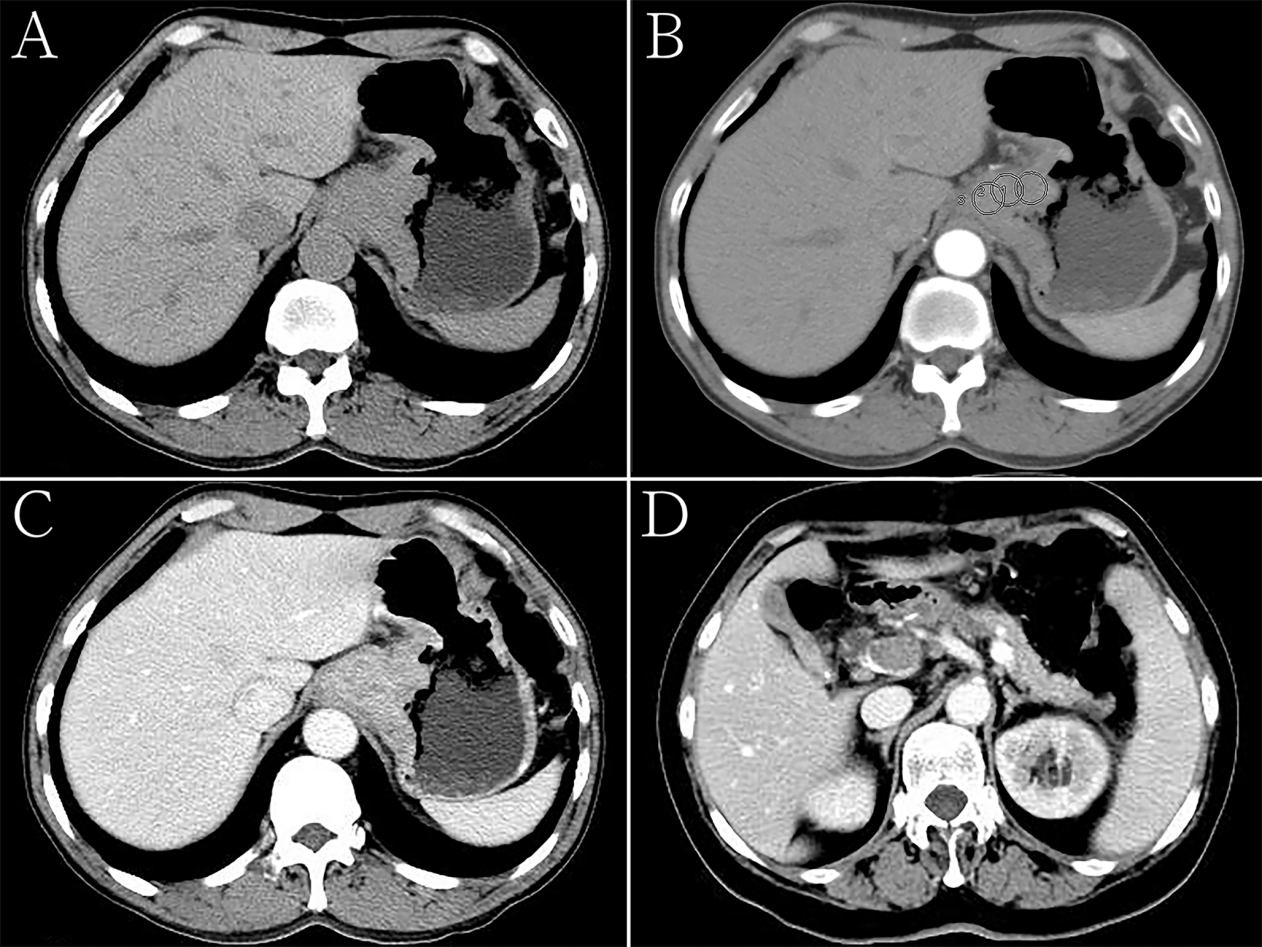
Hepatoid adenocarcinoma of the stomach in a 60-year-old woman. (A) Unenhanced computed tomography (CT) image of the stomach showing an intraluminal mass of homogeneous attenuation, with an irregular surface, in the cardia and fundus. The CT attenuation was approximately 52 Hounsfield units (HU). (B–D) Contrast-enhanced CT image showing moderate inhomogeneous enhancement of the mass, with the peak value observed in the portal phase. The thickest diameter of the mass was 4.7 cm. Ulceration was seen on the surface of the lesion. The Borrmann classification was type III. The CT attenuation in the arterial and venous phases were approximately 69 and 89 HU, respectively. Low-density tumor thrombi can be seen in the portal vein. (B) Arterial phase of the contrast-enhanced image, the CT attenuation were measured with regions of interests (ROIs) that were drawn manually, each with a diameter of about 15 mm^2^, while carefully avoiding vessels as well as necrotic and artifactual areas. The ROIs were measured at least three times and the average values were taken. (C, D) Portal phase of the contrast-enhanced image.
